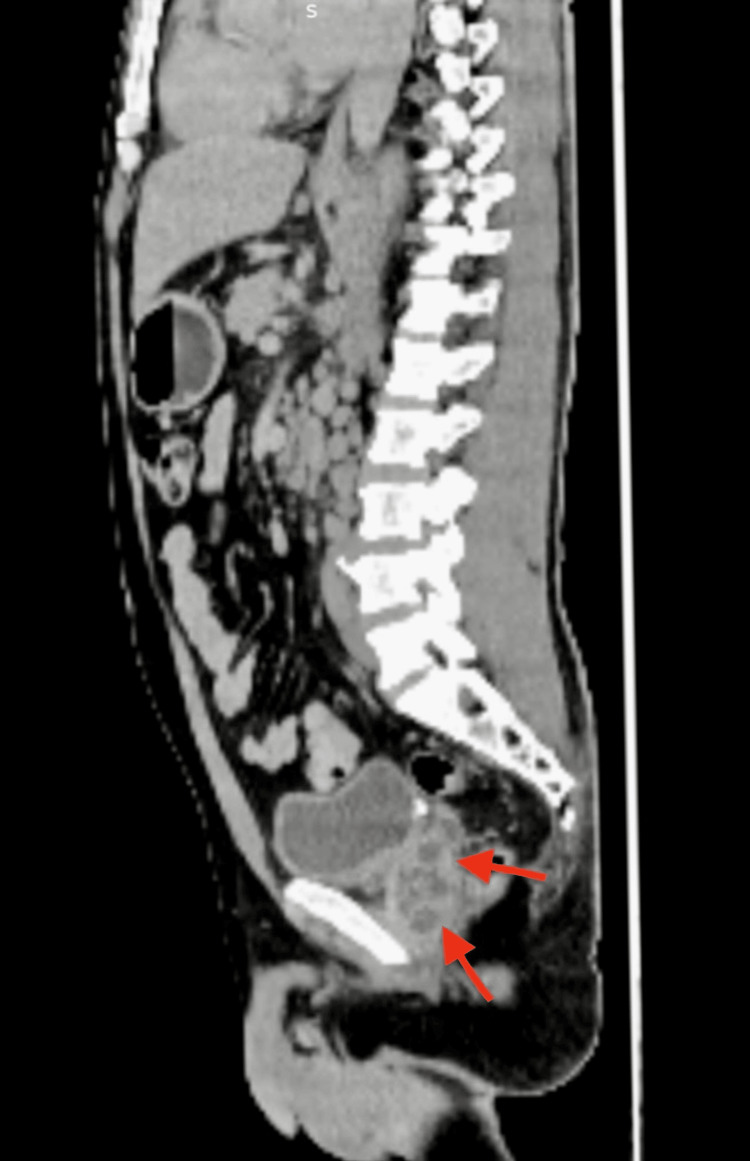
Sagittal section of CECT abdomen showing multiple hypodense lesions in the prostate (arrows) CECT: contrast-enhanced computed tomography
